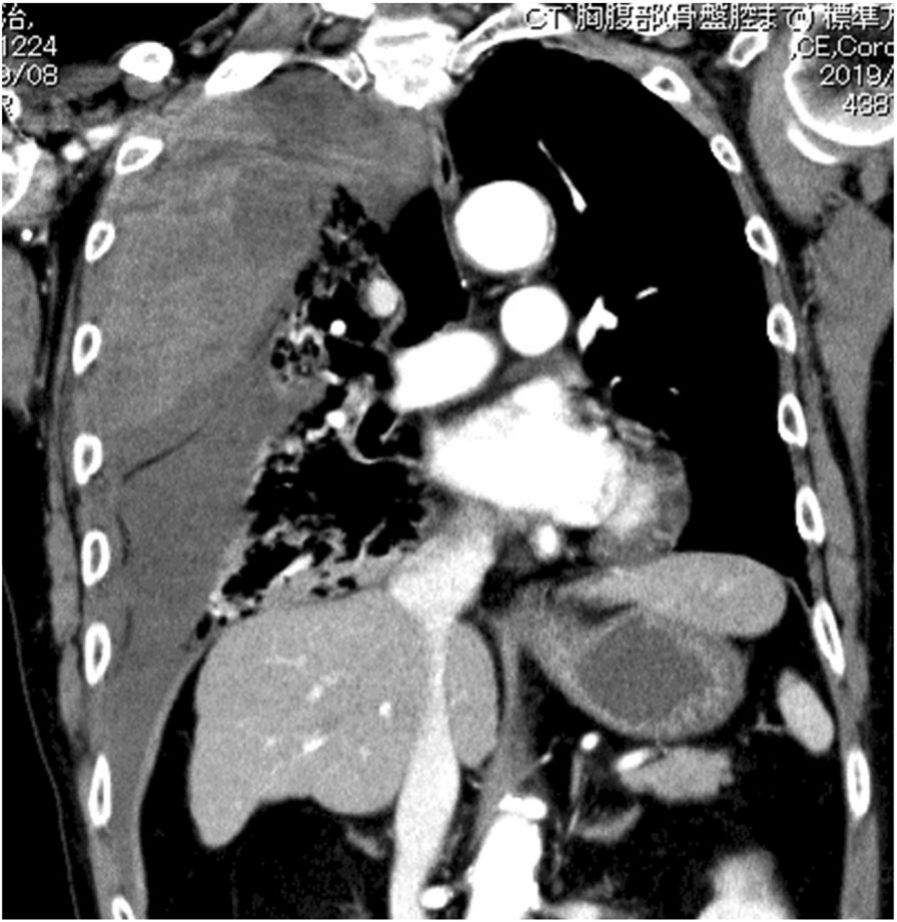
Chest computed tomography (CT) scan showing a hematoma in the extrapleural space
